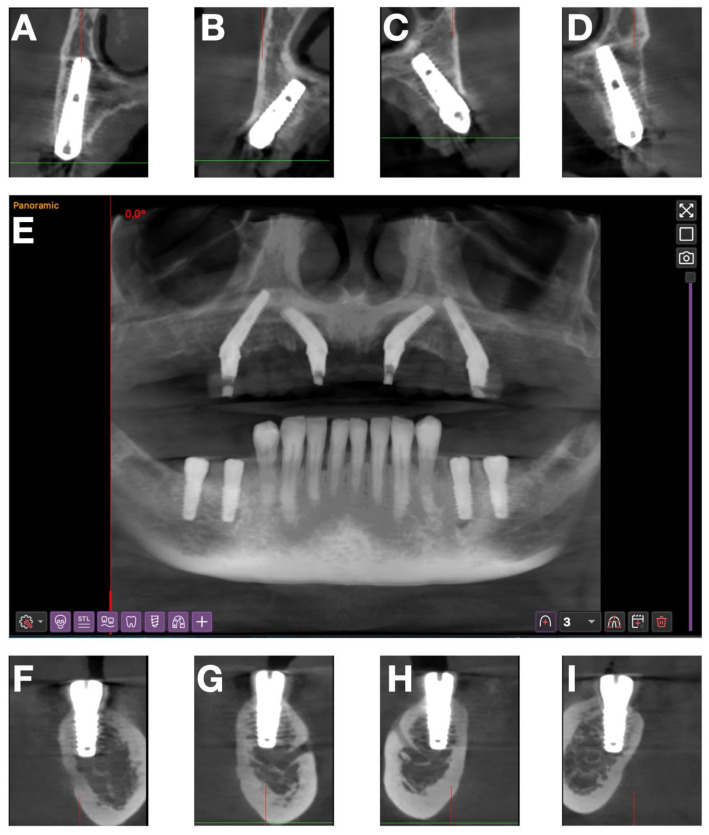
Postoperative CBCT: (A–D) sections at the level of maxillary implants 16, 12, 22, 26; (E) panoramic view; (F–I) sections at the level of mandibular implants 46, 45, 35, 36.
